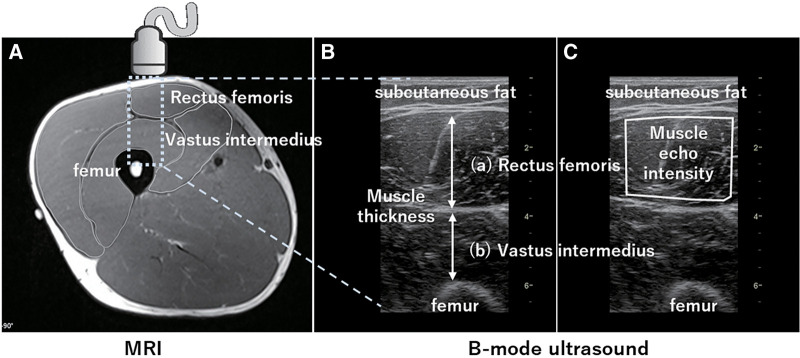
The measurements of muscle thickness and echo intensity using B-mode ultrasound (A) An axial MRI captured at the midthigh region. (B) A B-mode image taken at the same site. In this B-mode image, MT of (a) rectus femoris is measured as the distance between the muscle fascia, and MT of (b) vastus intermedius muscles is measured as the distance between the muscle fascia and bone interface. (C) The ROI for obtaining EI is set to avoid the surrounding fascia and the average EI within the ROI is calculated using image analysis software. A higher EI value (represented by white areas) indicates augmented fat deposition within the muscle. MT, muscle thickness; ROI, region of interest; EI, echo intensity
